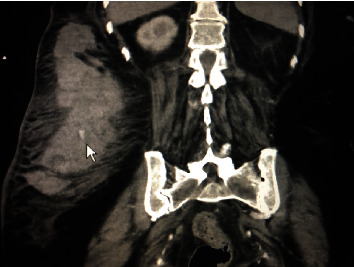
CT scan of the abdomen and pelvis for the second patient; the white arrow points towards a site of active extravasation (“blush”).
